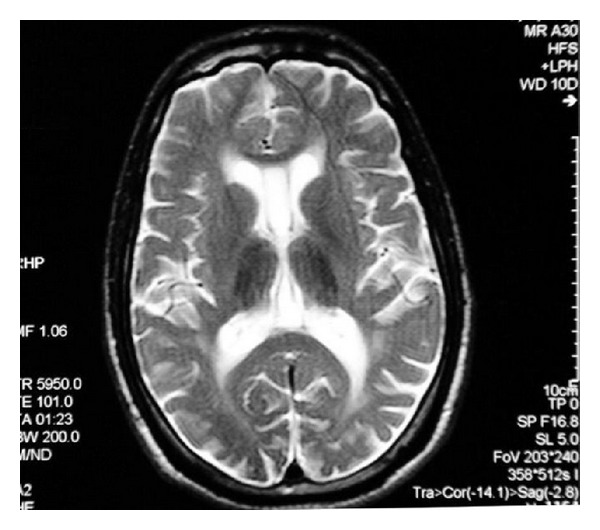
Interval axial weighted MR image of the brain following a six months of abstinence shows no significant interval change in the thalamic hypointensity or the periventricular white matter and posterior internal capsule hyperintensity, despite clinical improvement.
